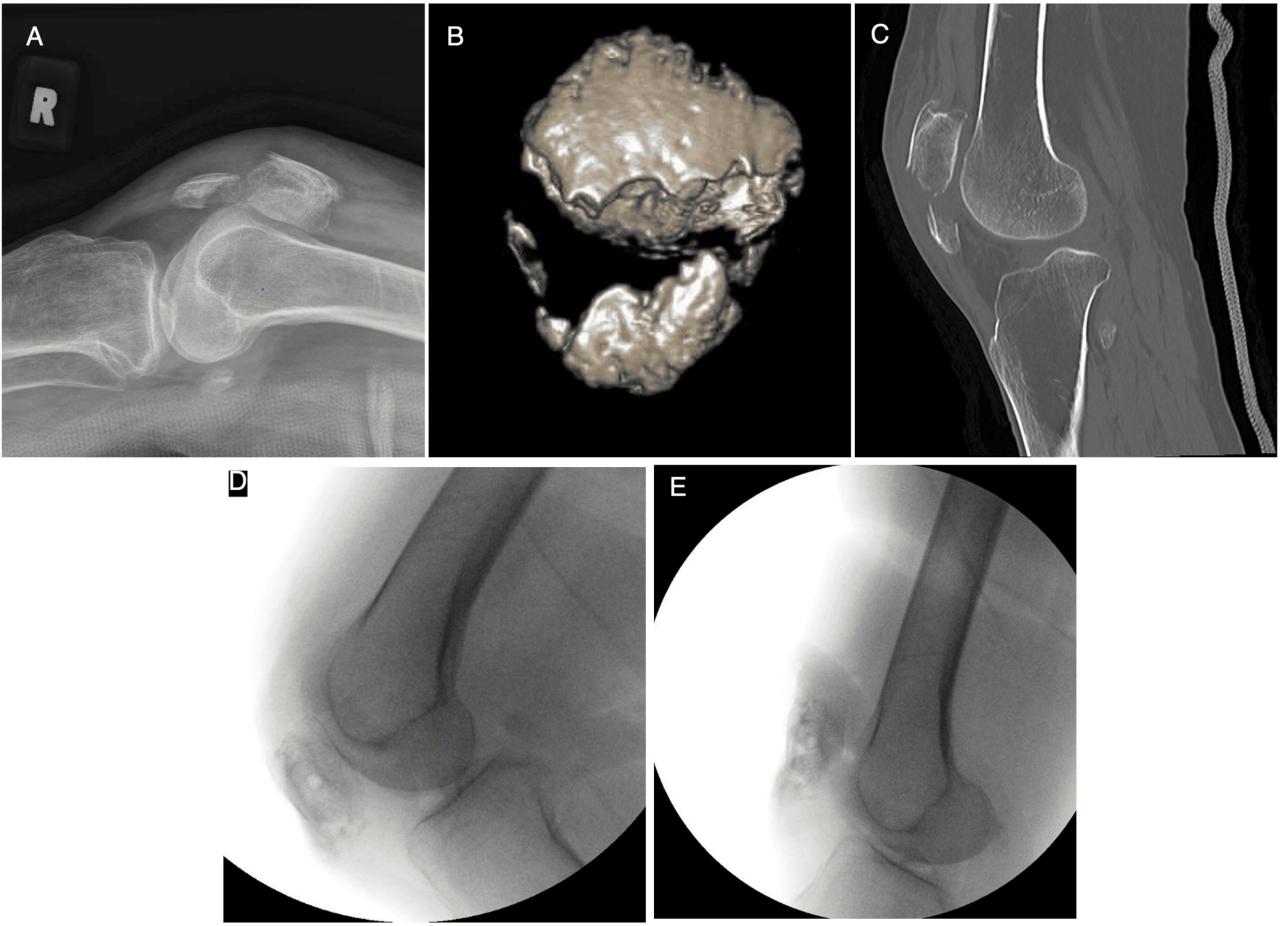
Pre-operative X-ray and computed tomography of right knee with 3D reconstruction A: Pre-operative plain radiograph of the right knee shows a fracture of the inferior pole of the patella with obvious displacement of the fractured end. B & C: Computed tomographic knee imaging with 3D reconstruction showing the comminuted small bone fragments in the inferior pole of the patella. D & E: Intraoperative imaging of the right knee showing good fracture reduction and stability with 90 degrees of flexion and full extension of the knee.
